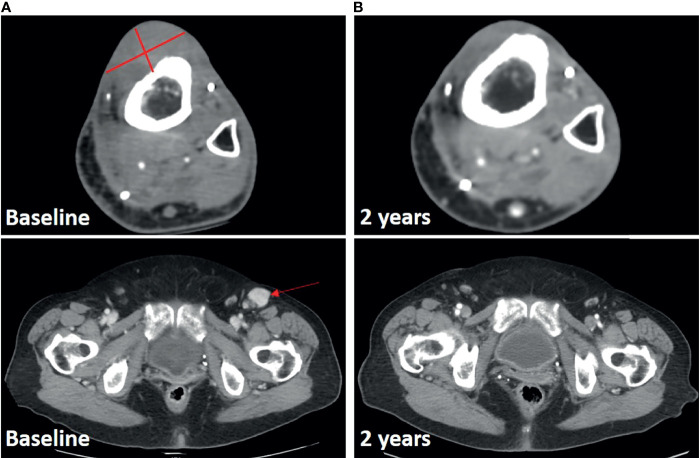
Response in Case 2 treated with ILI and pembrolizumab. (A), Baseline CT scan with lesions noted. (B), 2-year follow-up scan demonstrating ongoing response both in infused limb and at distant site.
